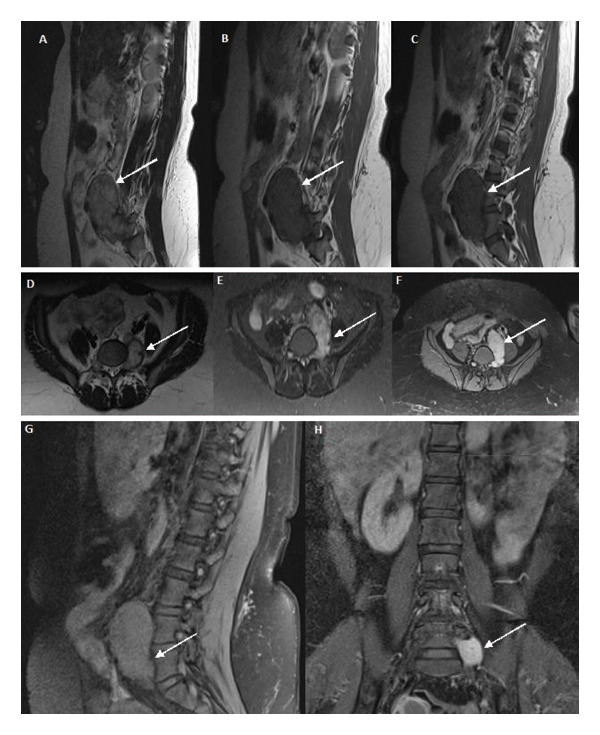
MRI spine (A), (B), (C) sagittal section; (D), (E), (F) axial section; (G) sagittal section; (H) coronal section. A 43 × 47 × 74mm well‐defined, T1‐isointense, T2‐hyperintense, lobulated lesion with homogeneous contrast enhancement, extending into the left L5–S1 foramen, and anterior to the left psoas muscle with anterior displacement of the left iliac vessels, suggestive of a left L5 spinal nerve sheath tumor or spinal schwannoma.
